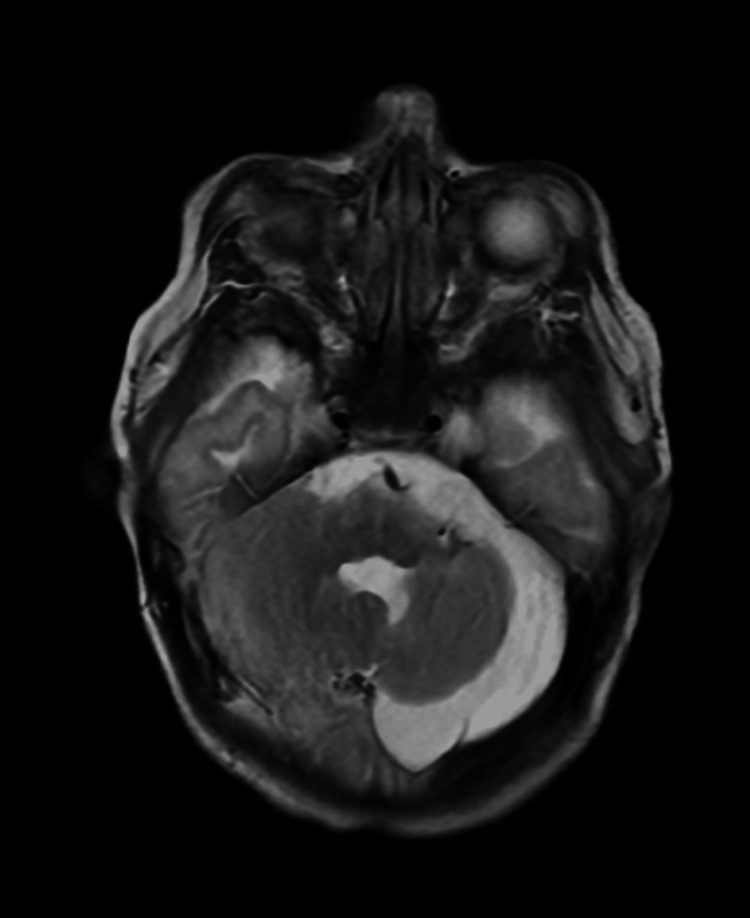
Brain MRI with contrast showed left cerebellar hypoplasia and an enlarged infratentorial subdural space. Cerebellar hypoplasia may contribute to potential developmental delays, balance difficulties, and neurological deficits in PHACE syndrome.
